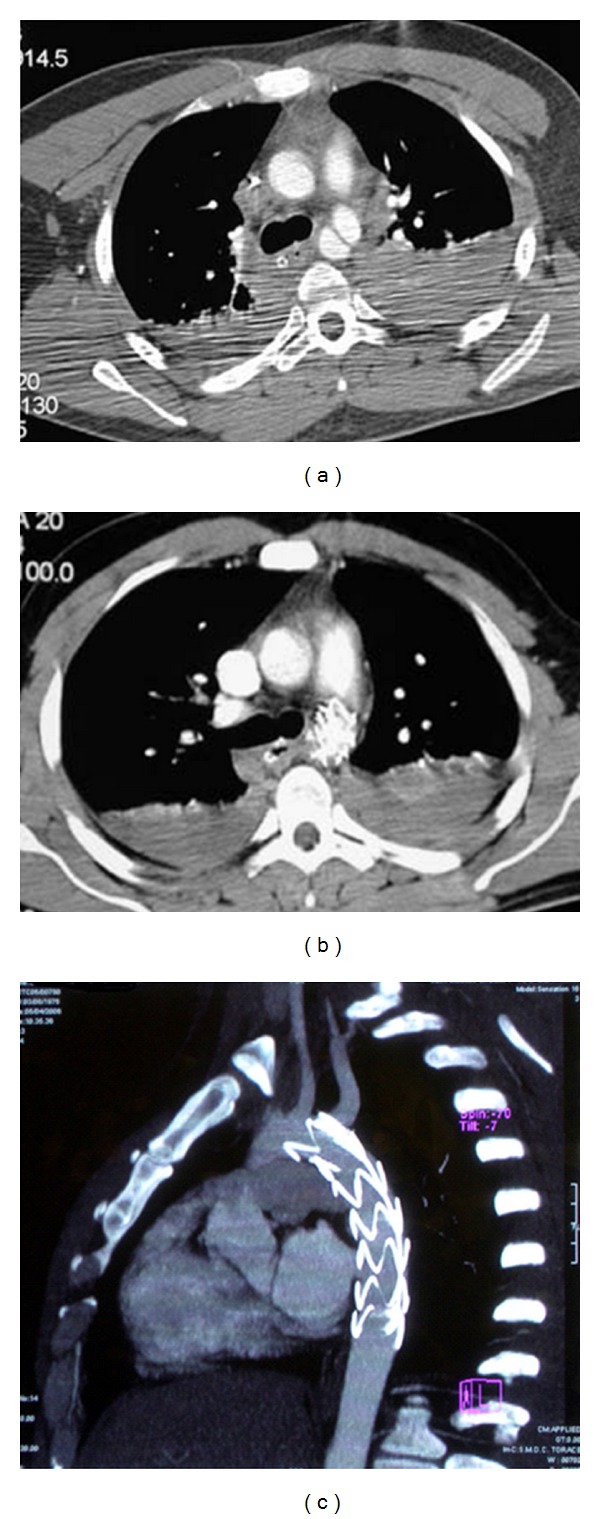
24 y. Patient-motorcycle accident. (a) Preoperative CT scan. (b) Postoperative CT scan. (c) CT scan control at 7 years.
